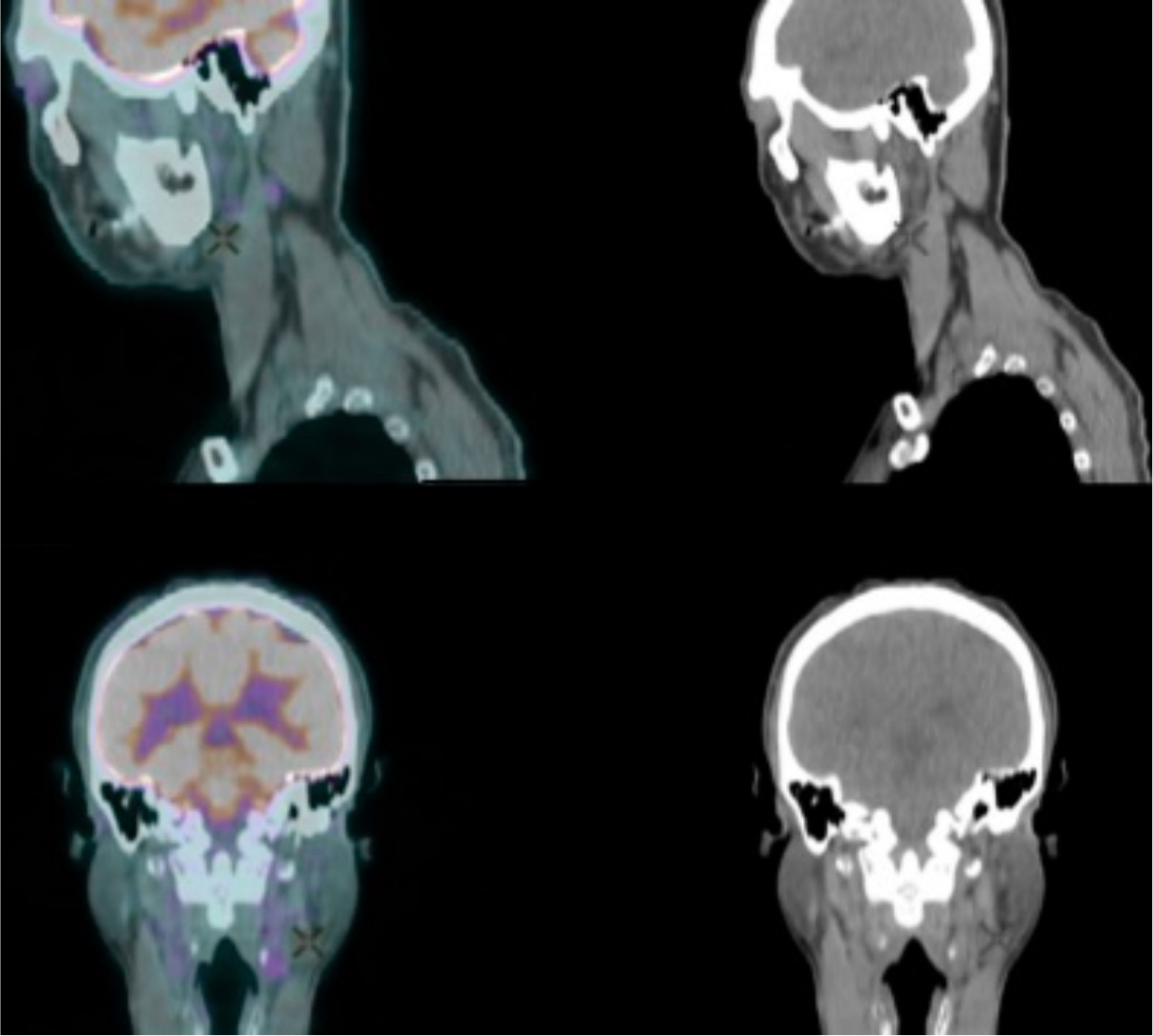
FDG PET shows hypometabolism in the concerned left upper neck lesion (cross).
